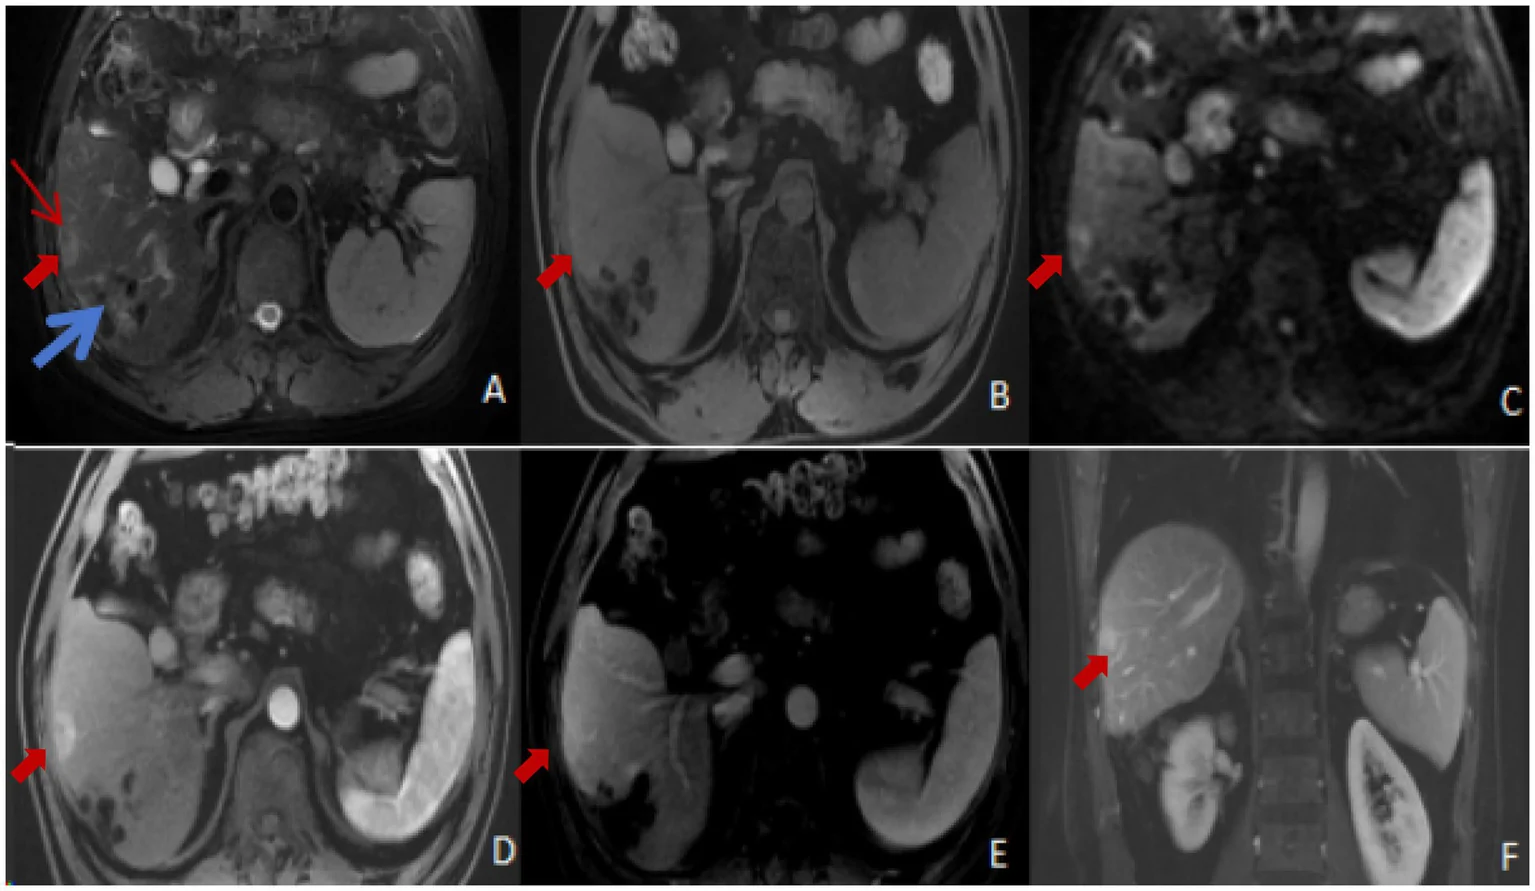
Enhanced MRI of the upper abdomen: (A) MR T2WI shows slightly high, uneven signal in the subcapsular area of the right anterior lobe, lower segment, near the surgical site. (B) T1WI is unclear. (C) DWI (*b* = 600 s/mm) shows high signal. (D) Significant enhancement in the arterial phase. (E) Mild enhancement in the portal venous phase. (F) Coronal view shows sustained enhancement in the delayed phase, higher than the liver parenchyma signal.
